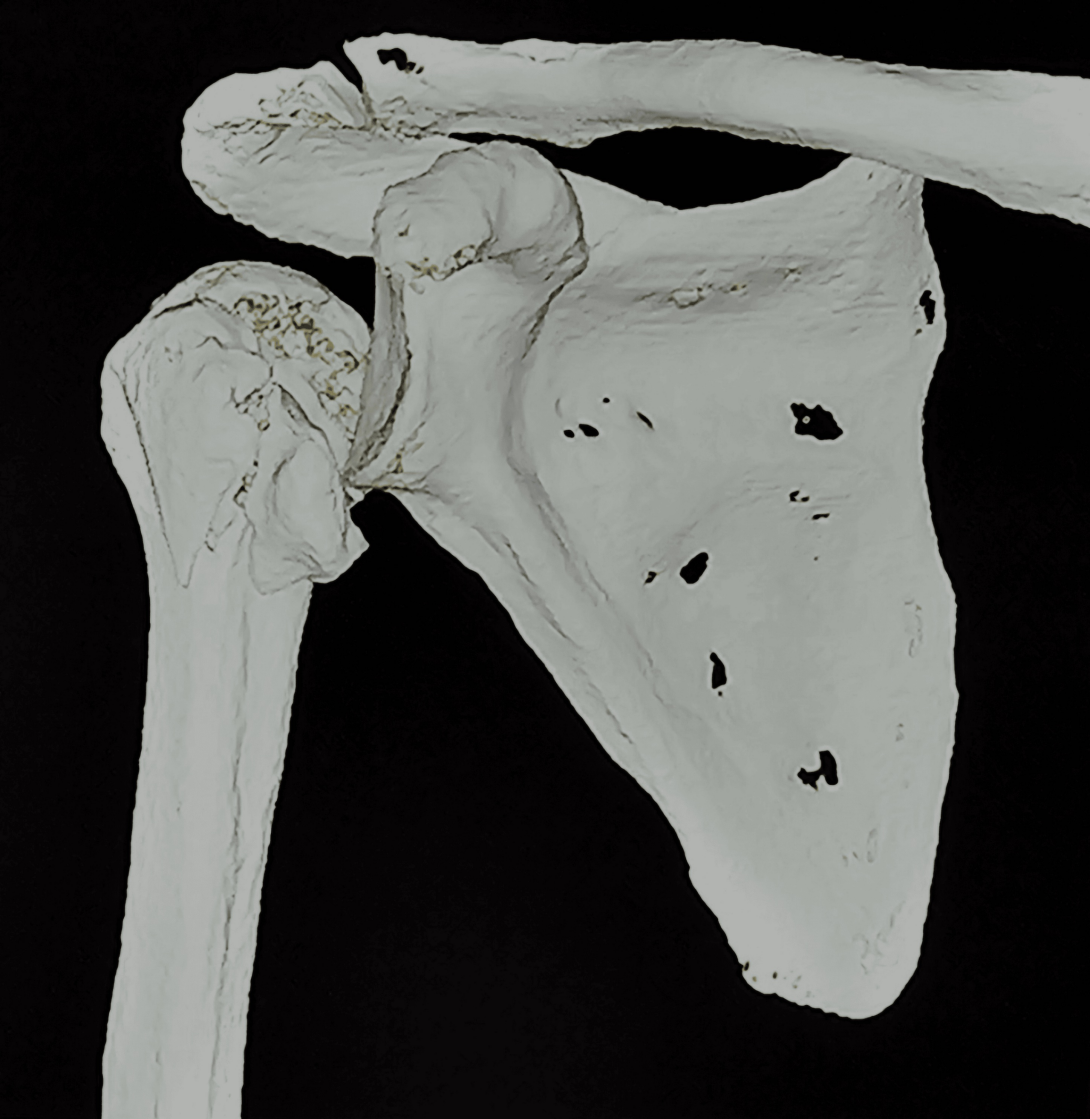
CT images with reverse Hill-Sachs lesion with GT and LT fractures. CT, computed tomography; GT, greater tuberosity; LT, lesser tuberosity
